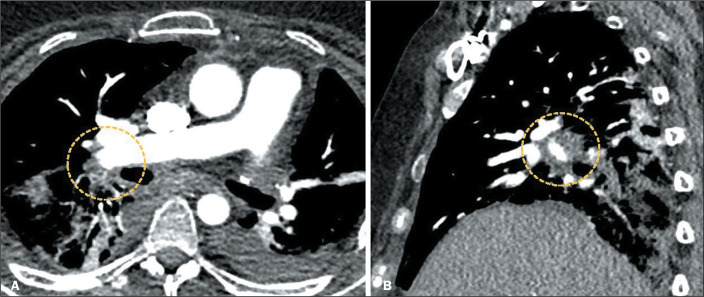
Pulmonary thromboembolism. CT angiography of the chest showing filling
